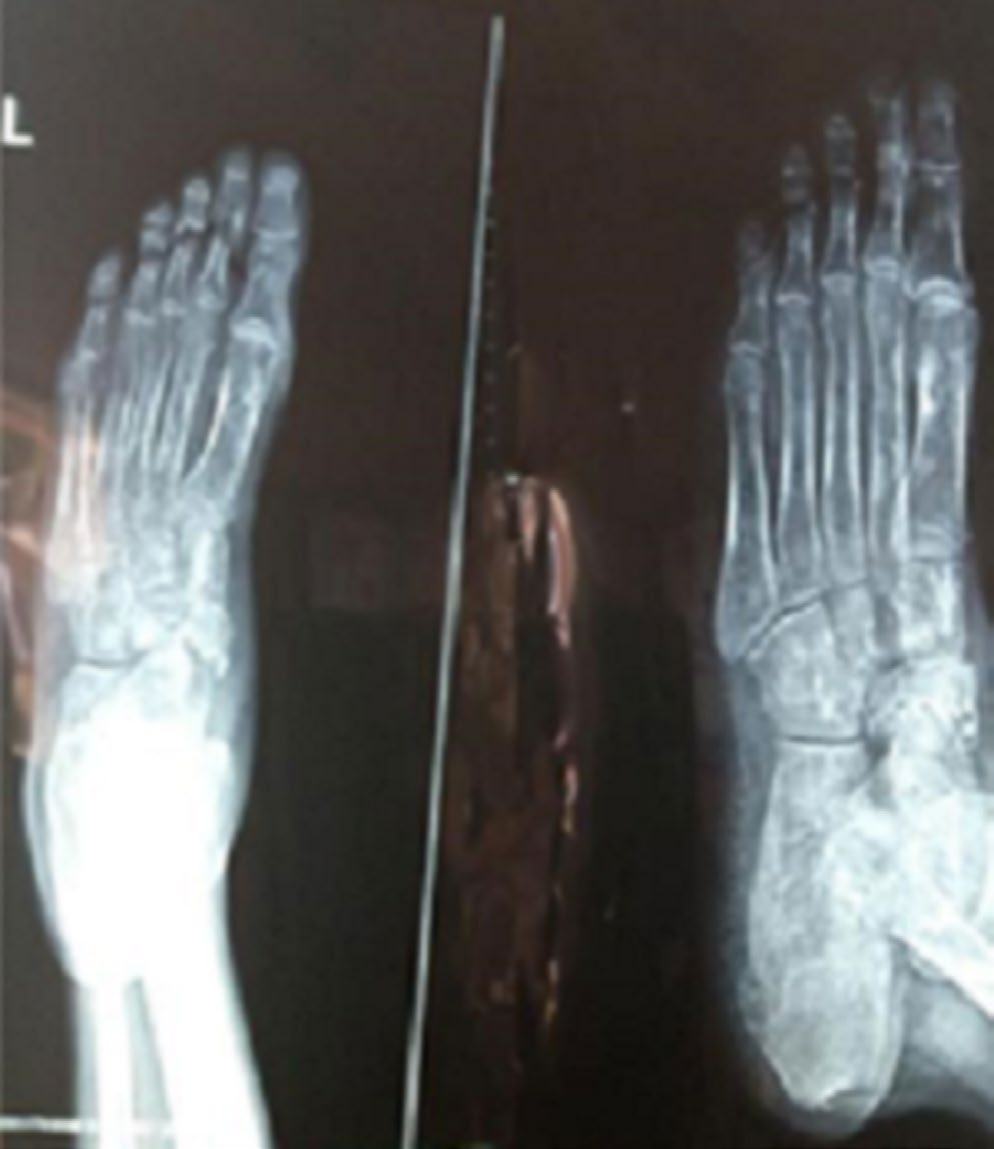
Foot x‐ray radiograph showing non‐erosive, non‐deforming arthritis of the ankle and mid‐foot joints.
